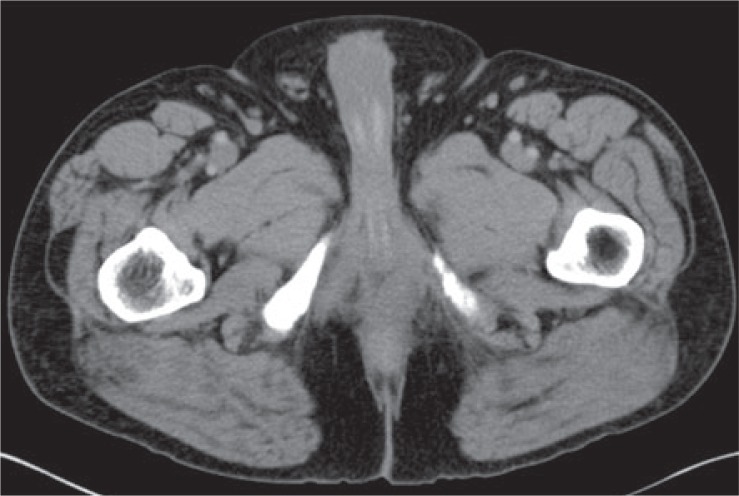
62 year old male with extraskeletal myxoid chondrosarcoma in the pelvis. Axial contrast enhanced CT showing locally recurrent tumor in the left perineum that is isointense to muscle.
